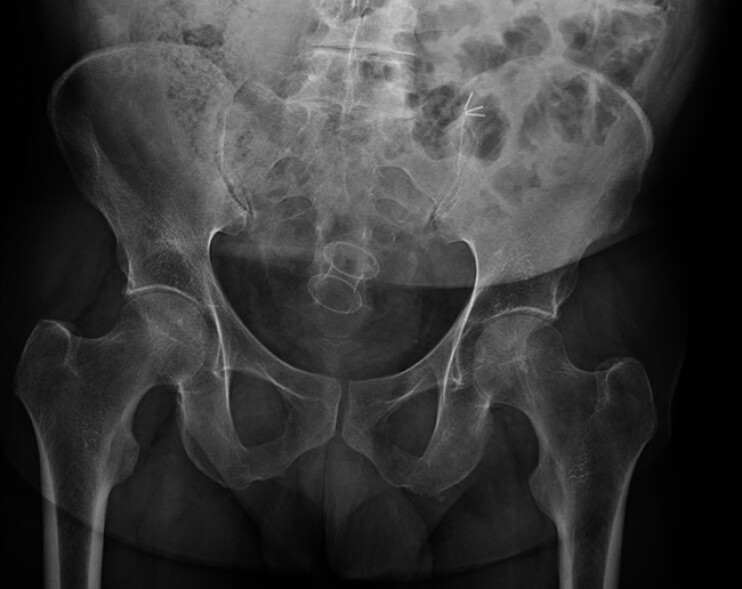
Pelvic radiography 1 week after LAMS placement showing an appropriate position and expansion. LAMS, lumen-apposing metal stent.
